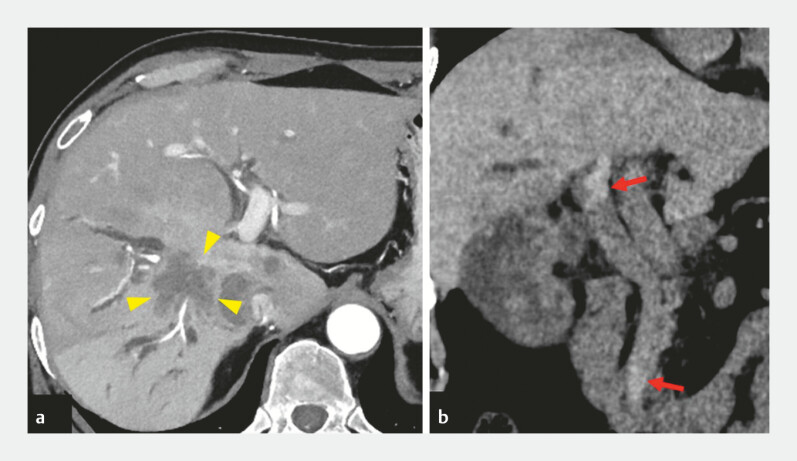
Computed tomographic images.
